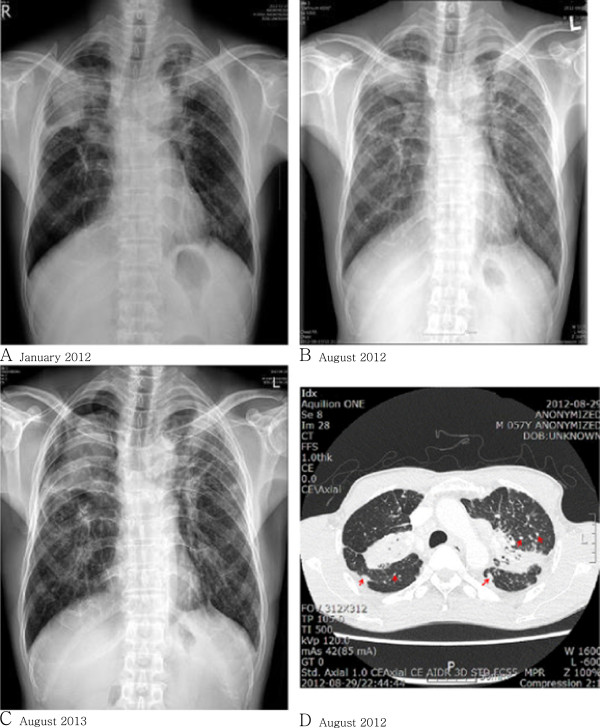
Chest X-ray. A. Pneumonitis or pulmonary tuberculosis in both upper lobes. A lung mass lesion in the right upper lobe was ruled out. B. Pneumoconiosis such as silicosis with progressive massive fibrosis (PMF). Slightly decreased densities around PMF were present since January 2012. A differential diagnosis for lung cancer, which is rarely considered, was performed. C. Pneumothorax, right. Underlying complicated pneumoconiosis, progressive massive fibrosis. HRCT. D. PMF, subpleural, and centrilobular silicotic nodules (arrows) are seen at both lung and pseudoplaque formation (arrow at pleural area) in the right lung.
